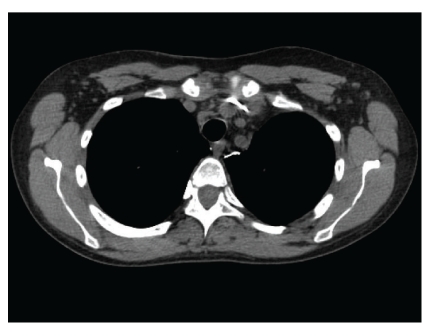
CT scan of chest posttreatment showed mediastinal nodes decreasing in size.
